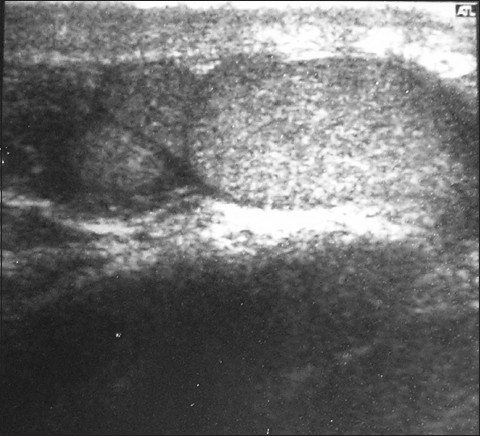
Longitudinal ultrasonographic scan of the right testis shows supernumerary testes at the lower pole
